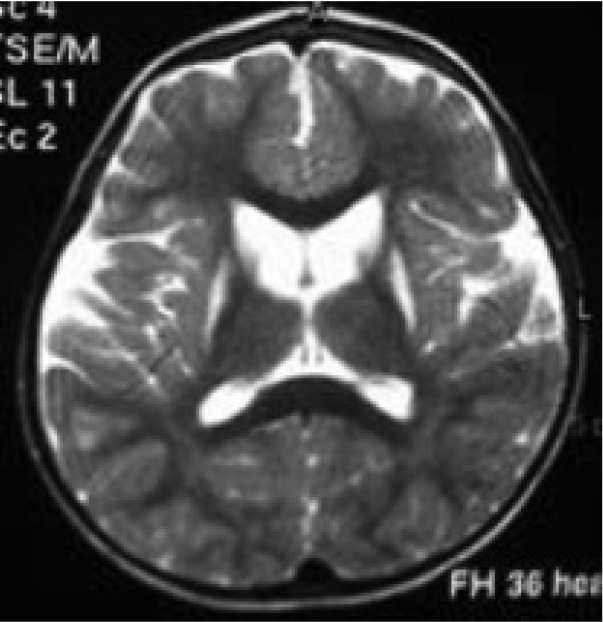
Axial T2 weighted MRI scan of patient 11 shows basal ganglia (putamen) hyperintensities
